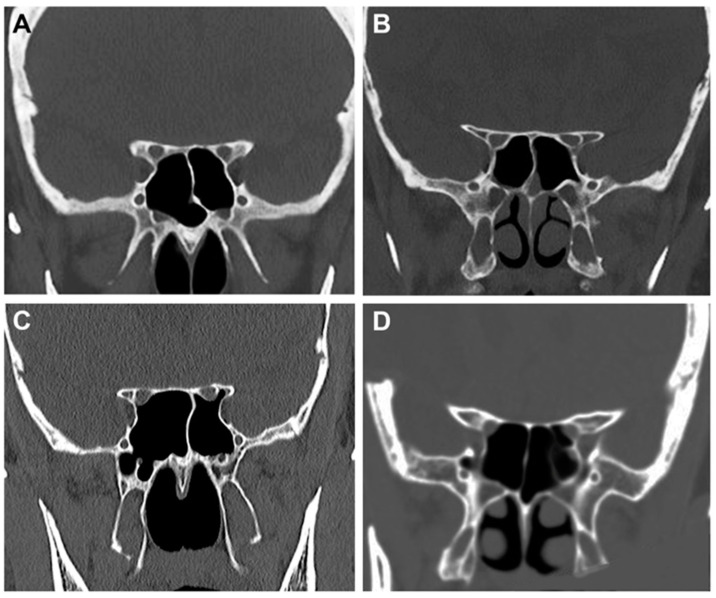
Coronal sections of the 4 variants of the optic nerve identified in the 200 CT scans according to DeLano’s classification [48]. (A) Type 1: the optic canal is located laterally to the sphenoid sinus, without any impression. (B) Type 2: the right optic canal is characterized by an impression on the lateral wall of the sphenoid sinus. (C) Type 3: both optic canals run into the sphenoid sinus. (D) Type 4: the right optic canal is characterized by the presence of Onodi cell or spheno-ethmoidal air cell.
